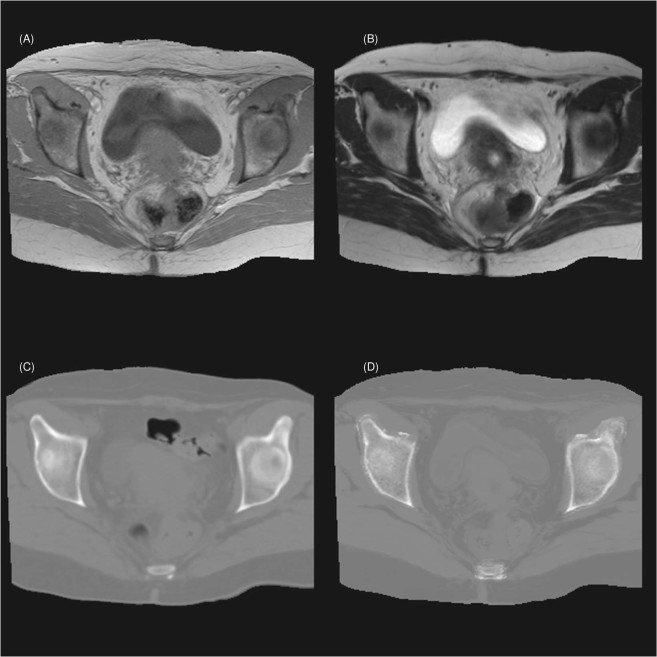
Axial views of the (A) MR_1_, (B) MR_2_, (C) reference-CT, and (D) generated pCT image at a given location of a target patient.
